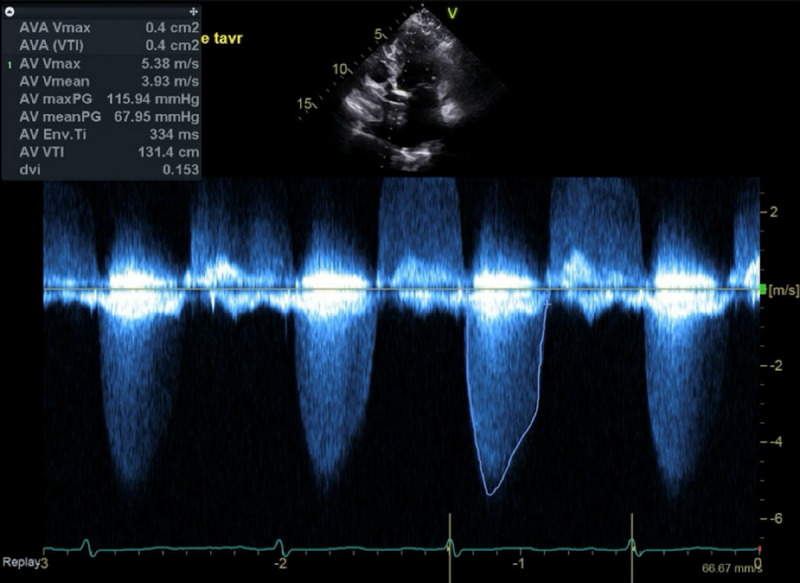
Continuous wave Doppler interrogation of aortic valve demonstrating mean gradient 68 mm Hg, Vmax 5.38 m/s, AVA 0.4cm^2^, DI 0.15 consistent with severe aortic stenosis.
